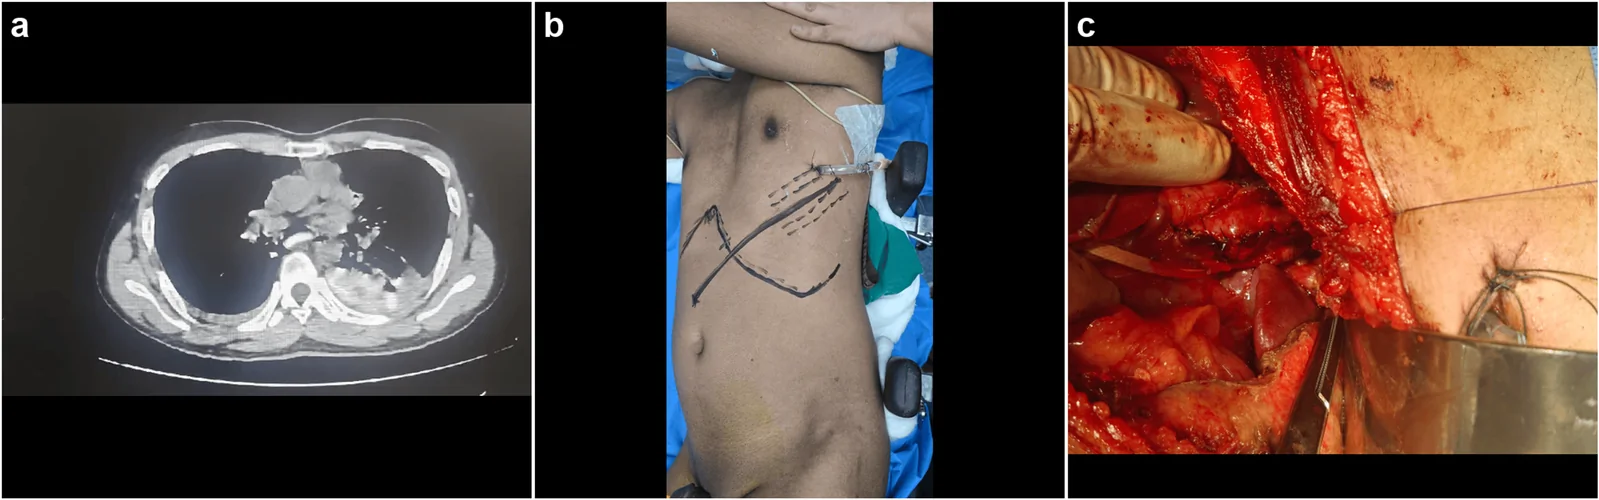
Case 3 Images (a) Axial oral-contrast computed tomography showing leak into the left pleural cavity. (b) Marked thoraco-abdominal incision. (c) Intra-operative view of the repair.
